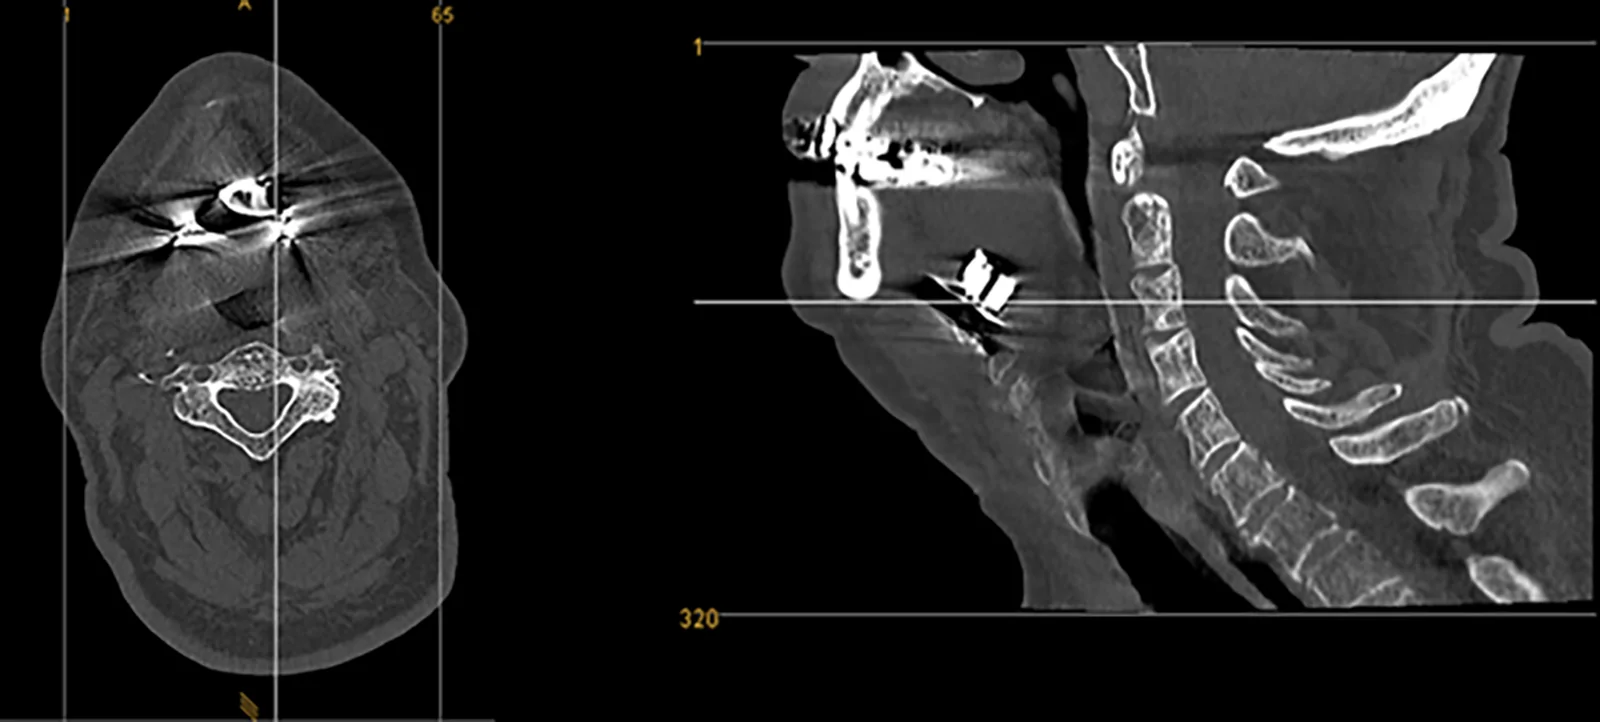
CT (axial and sagittal planes). biHGNS implant position and moderate artifacts.
